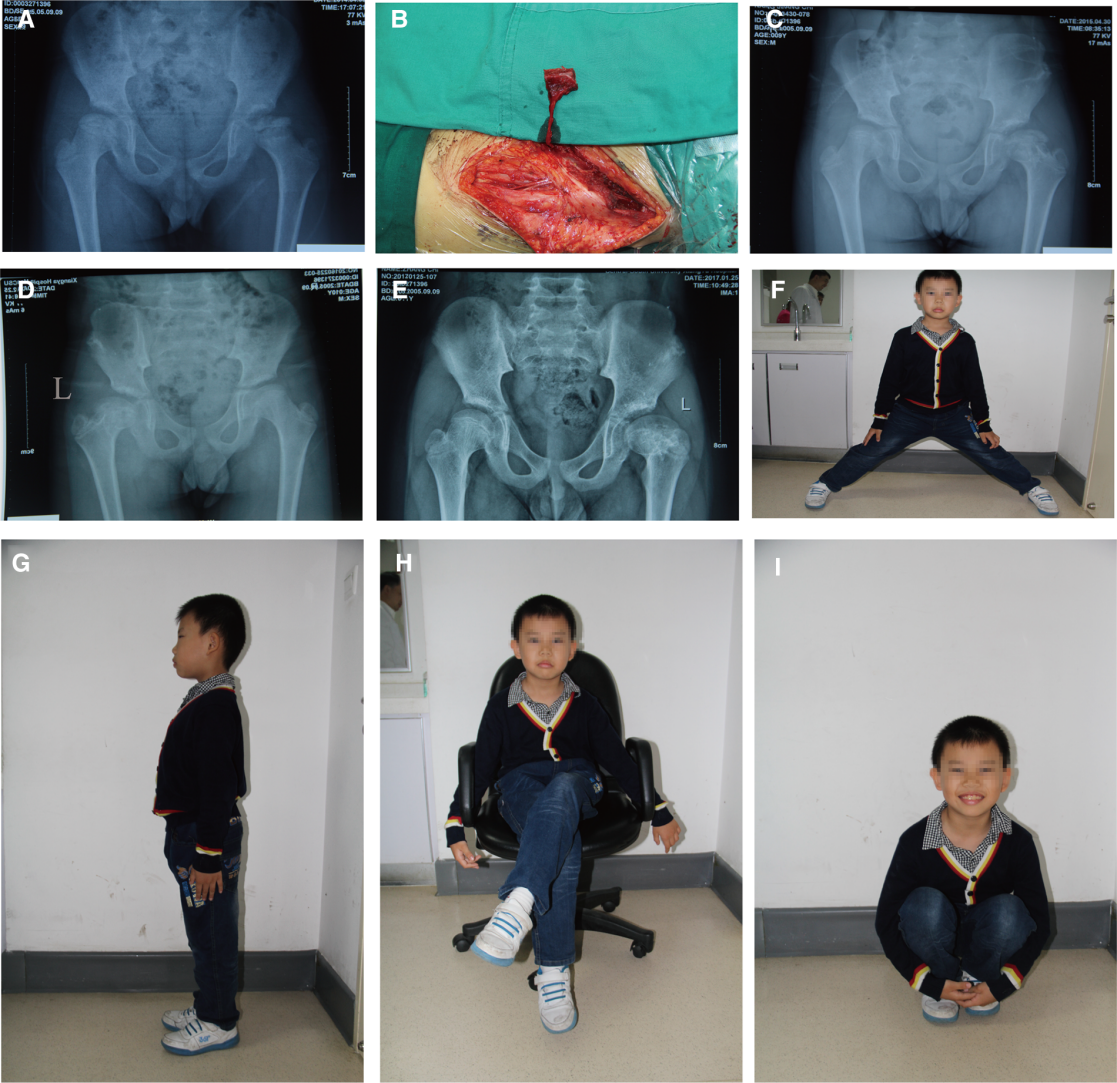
An 8-year-old boy underwent a pedicled iliac bone flap based on deep circumflex iliac vessels to treat LCPD of the left side (lateral pillar grade C). (A) Preoperative x-ray film. (B) The pedicled iliac bone flap was harvested. Postoperative x-rays at (C) 12 months, (D) 24 months, and (E) 36 months showed no collapse of femoral heads or narrowing of the hip joint spaces. Hip (F) abduction, (G) Extension, (H) external rotation, and (I) flexion showed good recovery. LCPD, Legg–Calvé–Perthes disease.
